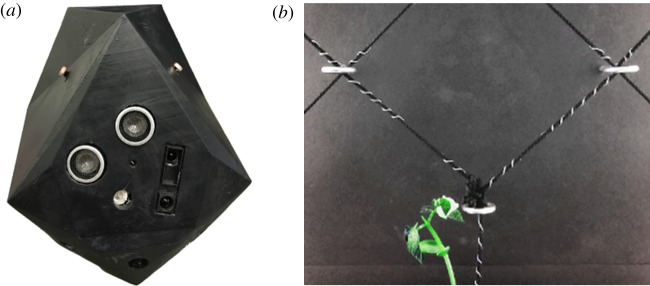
Preliminary mechatronic prototype and experiment for testing the successful IR sensing, unsuccessful ultrasonic sensing, and unsuccessful capacitive sensing. (*a*) Prototype incorporating both IR and ultrasonic proximity sensors, for comparison of accuracy. (*b*) Test of capacitive sensing, via tin wire wound onto diagrid filaments.
